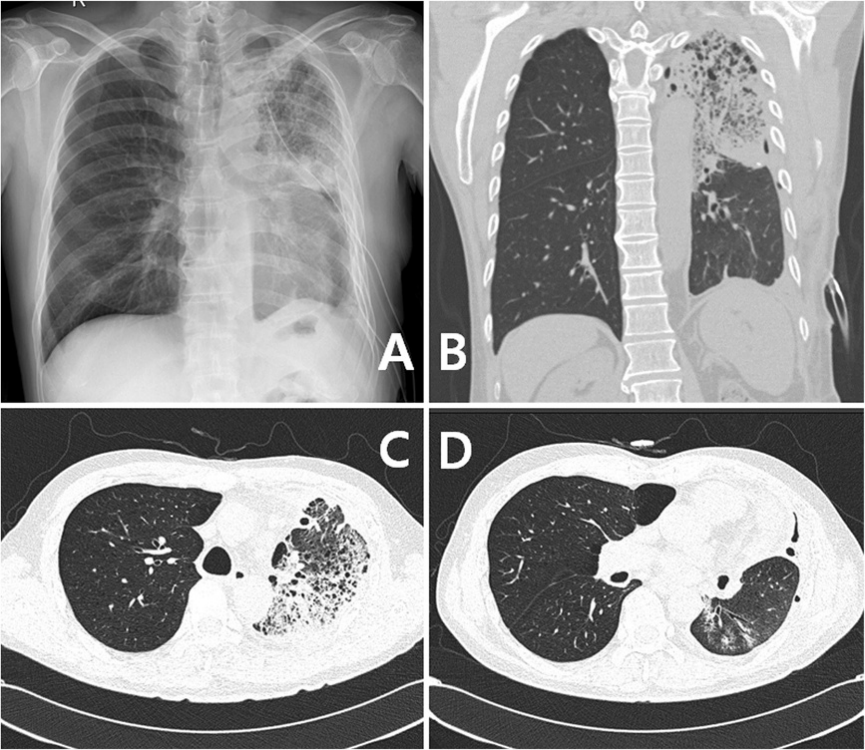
Images of acute pneumonitis. A follow-up chest X-ray shows increasing extents of peribronchial consolidations and infiltrations in the left upper lung (A). A chest computed tomography reveals extensive parenchymal consolidations in the whole left lungs, air-fluid levels within the emphysematous bullae, and multiple reactive mediastinal lymph nodes; all of these findings indicated a suspicious of pneumonia (B, C, D).
